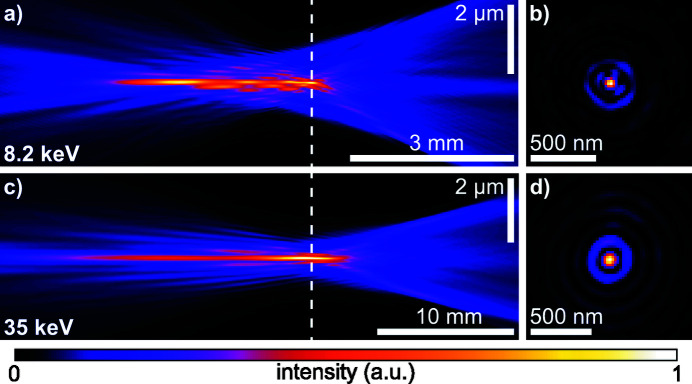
Characterized X-ray beams with spherical aberration. (*a*) Horizontal beam caustic at 8.2 keV. (*b*) Intensity distribution in the plane with highest peak intensity, marked by the dashed line in (*a*). (*c*) Horizontal beam caustic at 35 keV. (*d*) Intensity distribution in the plane with highest peak intensity, marked by the dashed line in (*c*).
